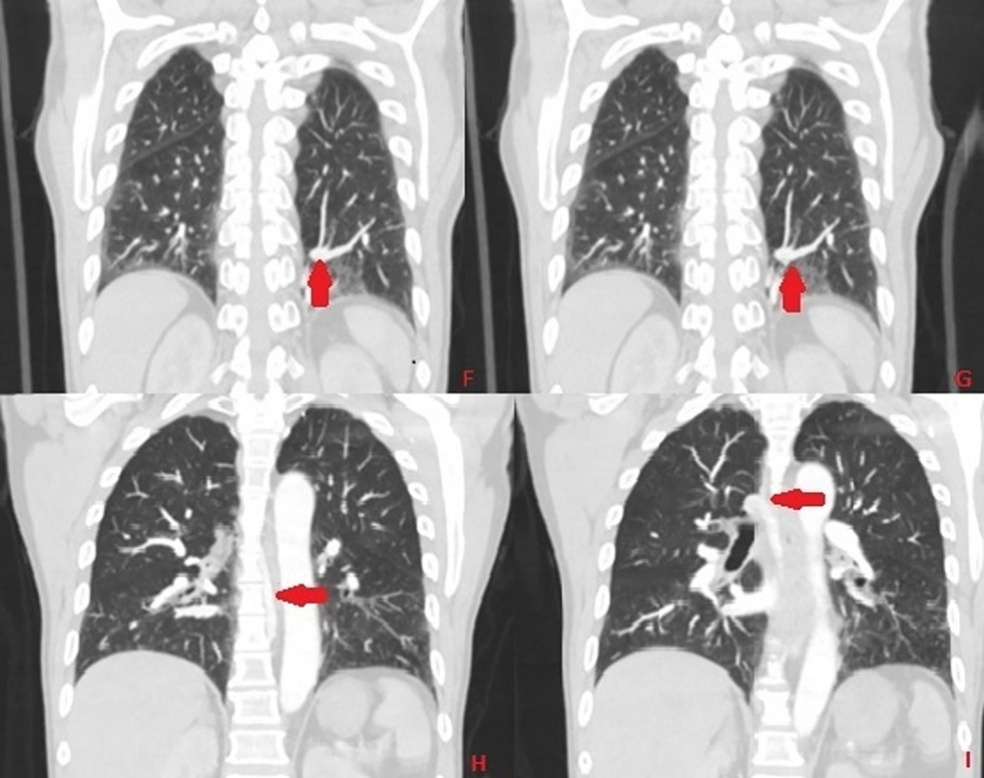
CT of the chest with contrast in the frontal plane and lung window with red arrow demonstrating the course of anomalous pulmonary vein arising from intralobar sequestration (Image F and G) coursing on the side of the aorta (Image H) ultimately joining the azygos vein (Image I)
